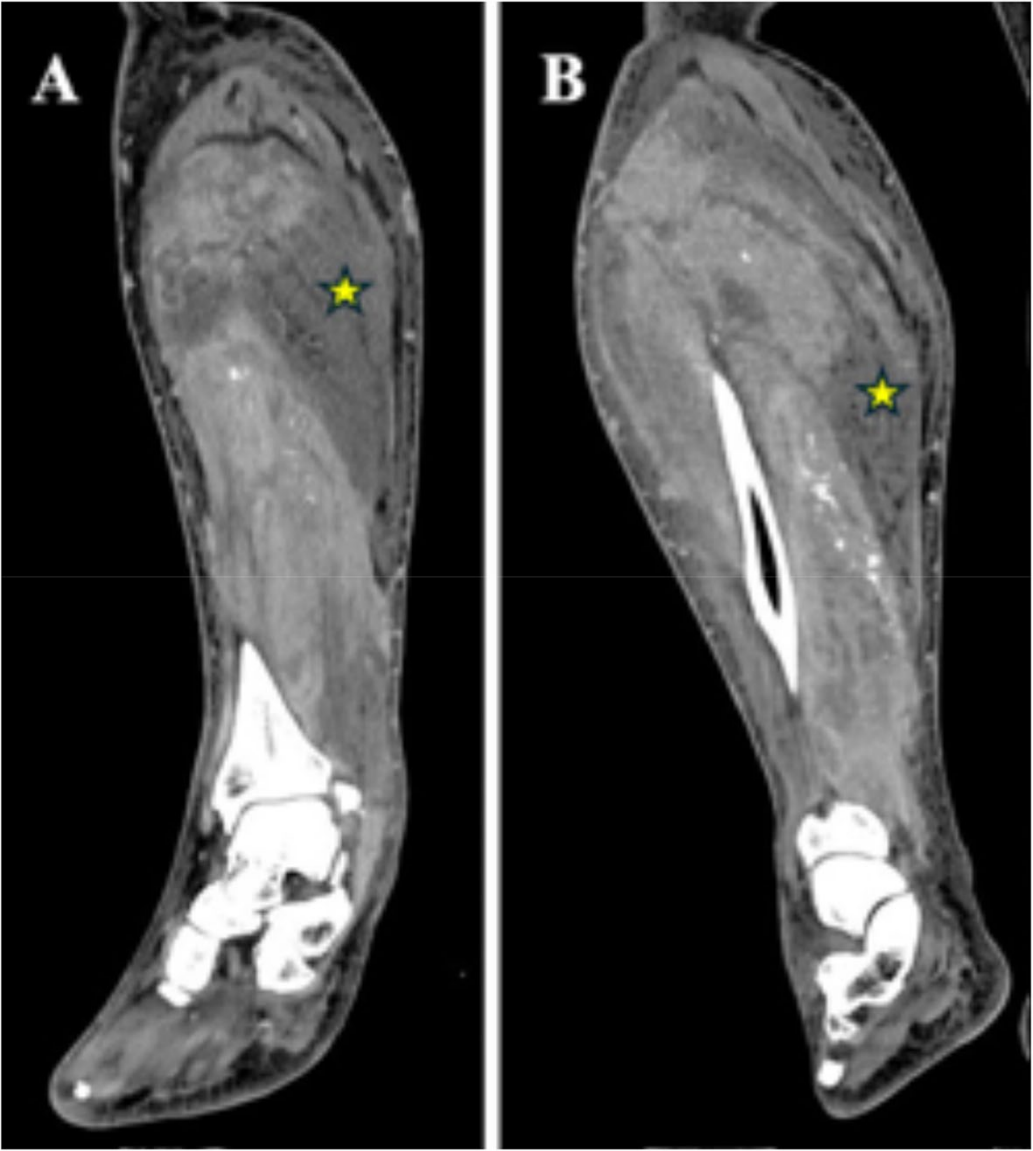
A, B Coronal CT images of the right lower extremity with IV contrast showing significant abnormal hypodensities within the right gastrocnemius muscle representing myonecrosis (stars)
